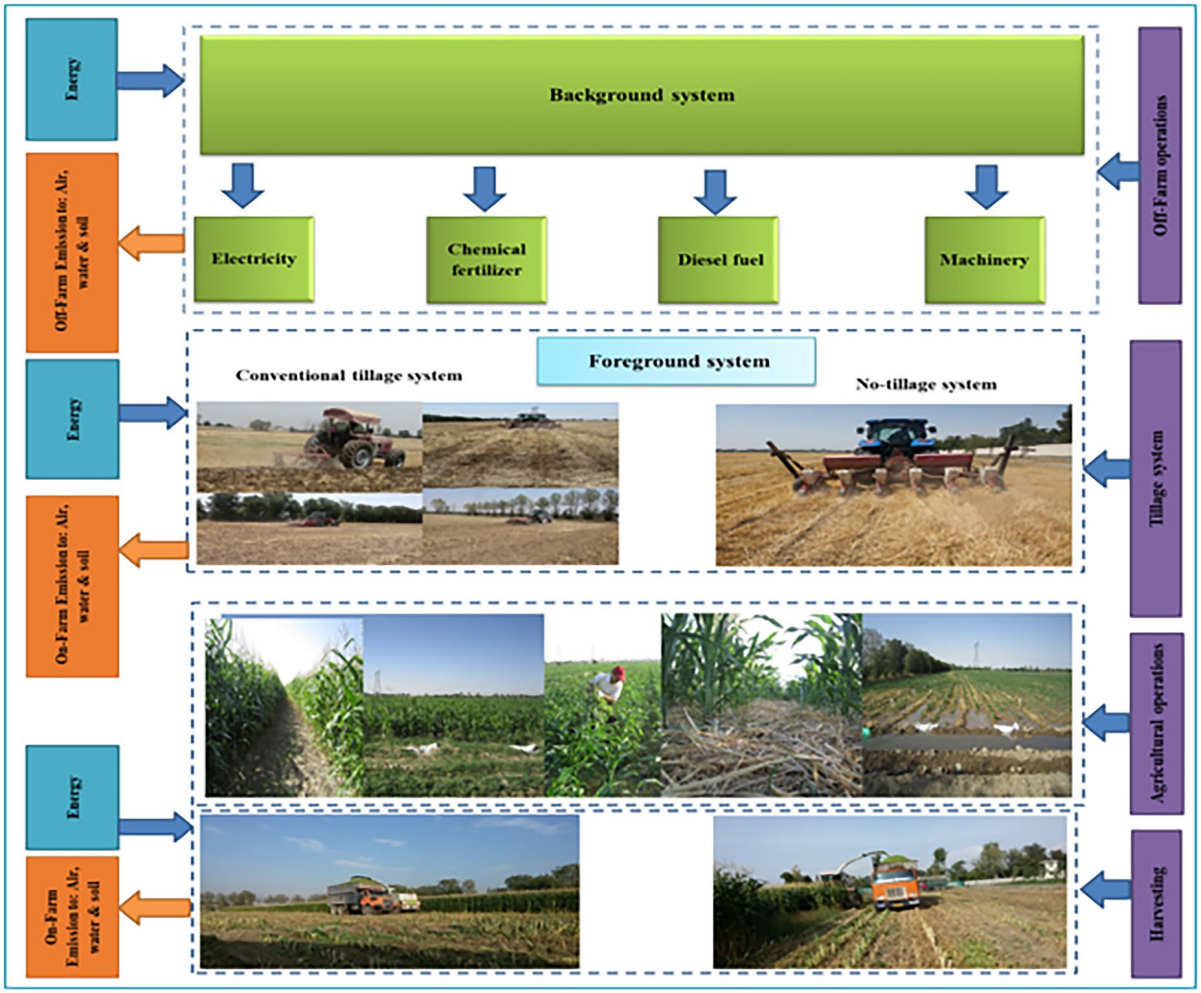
System boundaries of corn silage production under CT and NT systems
